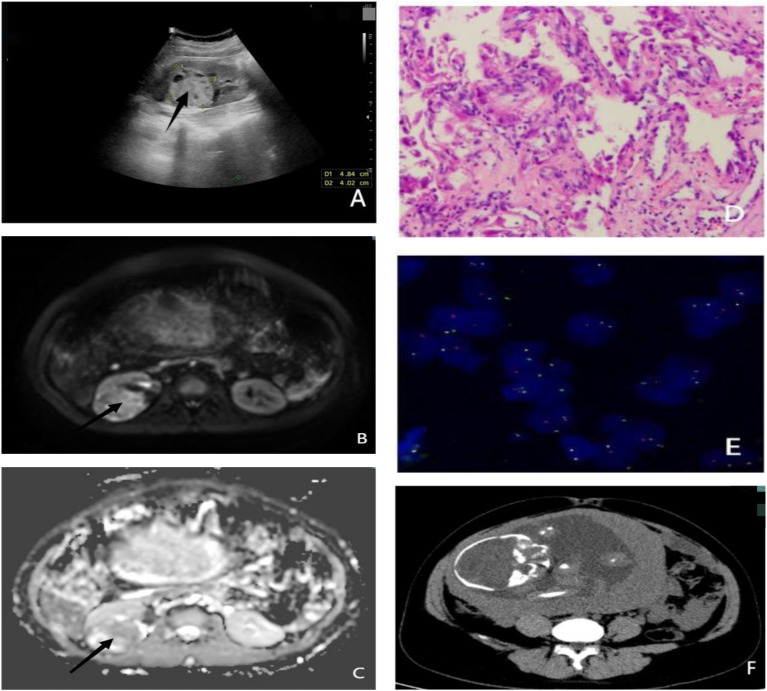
(A) Ultrasound showing a hyperechoic cystic-solid tumor of the right kidney measuring 4.8 × 4.0 cm. (B) MRI ADC sequence of renal tumor. (C) MRI DWI sequence of renal tumor. (D) Pathological section: cytoplasmic hyaline in most of the cells, eosinophilic nucleoli in a few cells, and gravel body-like calcifications. (E) TFE3 (Xp11.2) gene breakage detection probe revealing broken TFE3 (the ratio of break signals is 43%, exceeding the threshold of 10%). (F) Lower abdomen CT showing the 29-week fetal morphology. ADC, apparent diffusion coefficient; DWI, diffusion-weighted imaging.
